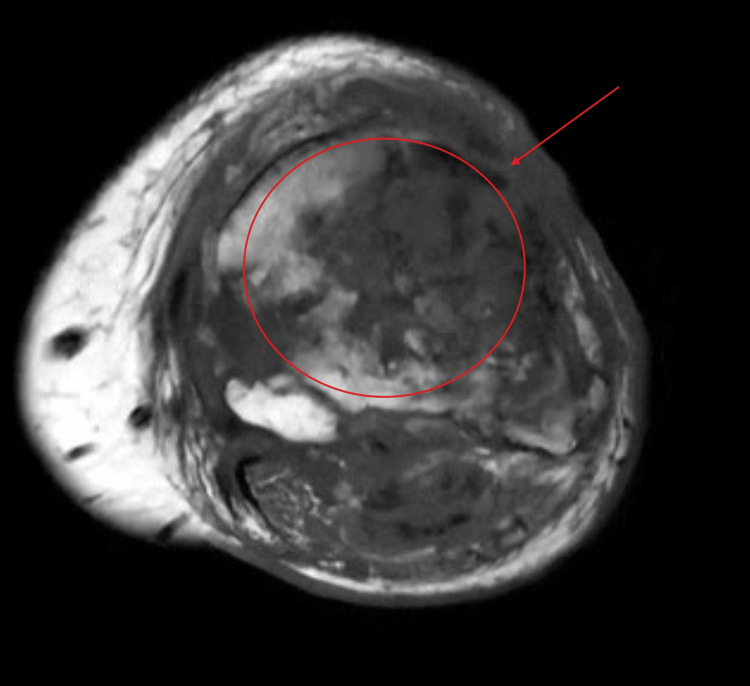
Cross-sectional MRI depicting extensive intramedullary tumor burden in osteosarcoma (current)
